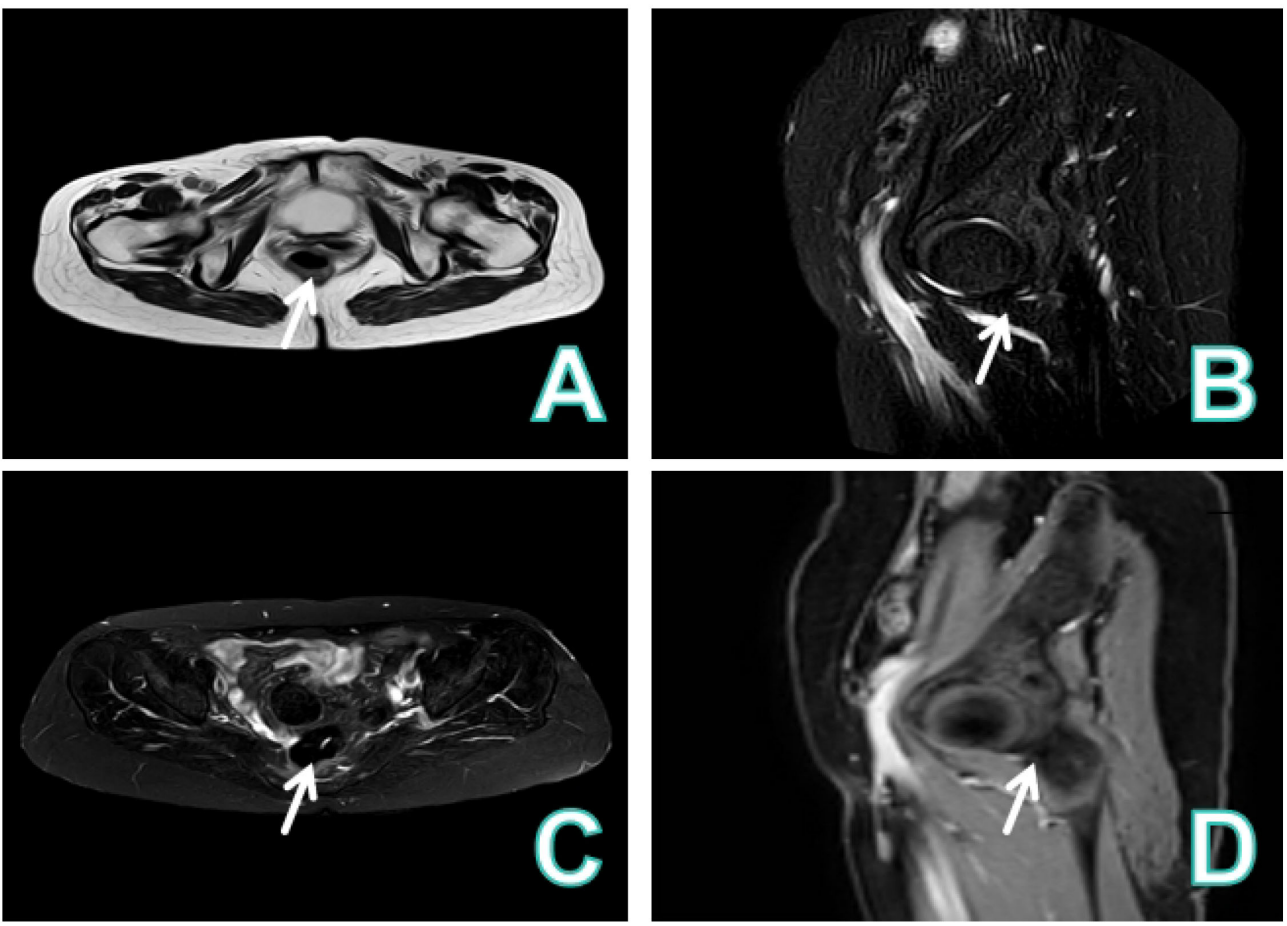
Contrast-enhanced MRI image. (A) The vaginal wall was thickened and enhanced, and the posterior fornix showed a mass with long T1 and slightly long T2 signals. (B-D) The lesion was close to the posterior uterine wall and had a clear boundary with the rectum. Diffusion of the lesion was restricted, and enhanced scan revealed heterogeneous enhancement with obvious peripheral enhancement, making abscess a possible consideration.
